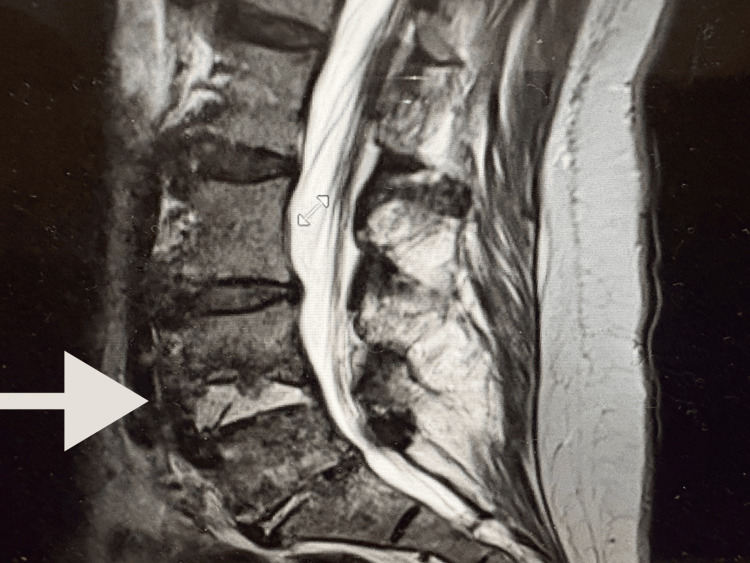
T2-weighted MRI of the lumbar spine showing L4-L5 vertebral osteomyelitis and discitis with Modic type 1 changes, along with a paravertebral phlegmonous process including small bilateral psoas abscesses and surrounding myositis
